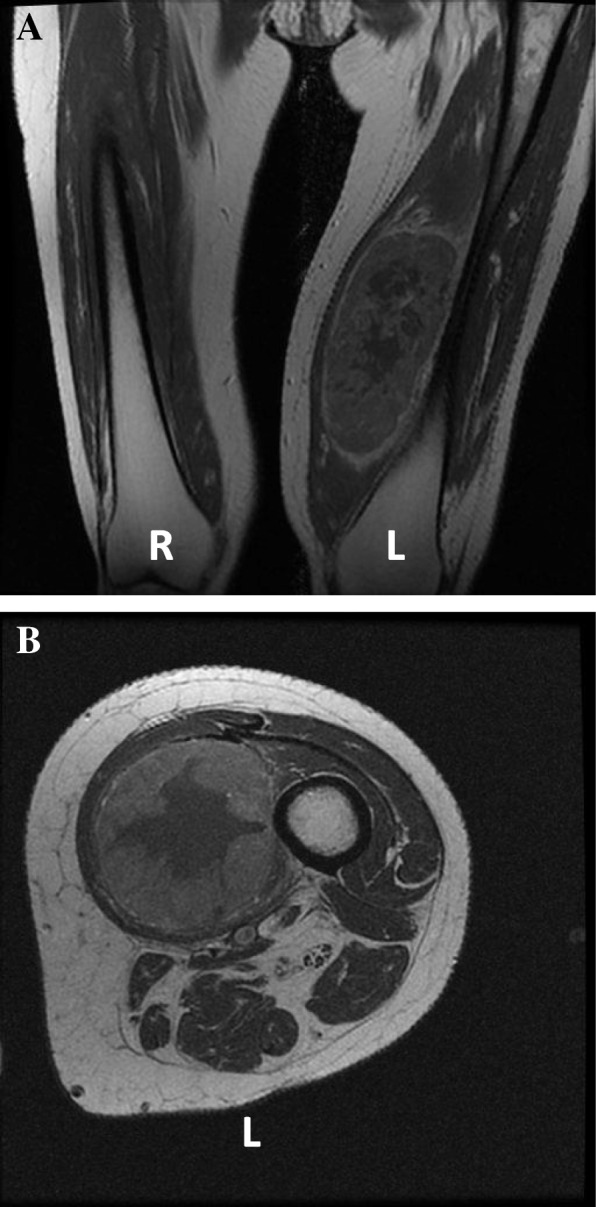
Magnetic resonance imaging investigation of left thigh mass. a Coronal magnetic resonance image of thighs. b Cross-sectional image of left thigh
